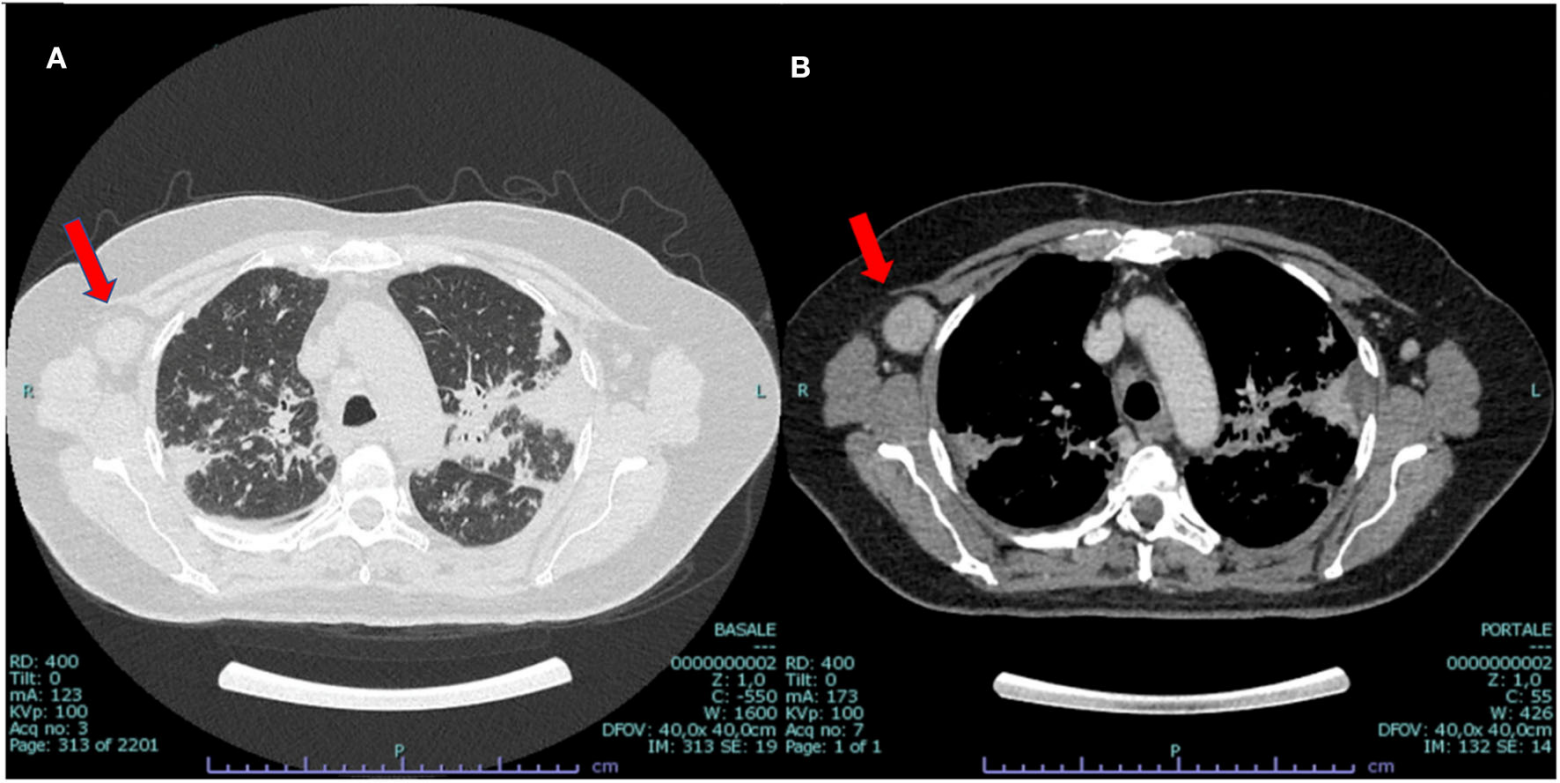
CT scan at presentation: bilateral parenchymal infiltrates (A) and mediastinal hilar lymph node enlargement (B), suggestive of sarcoidosis but also compatible with malignant origin. Enlarged vascularized pathologic (3 cm) axillar node detected in the right axillary region (red arrow).
